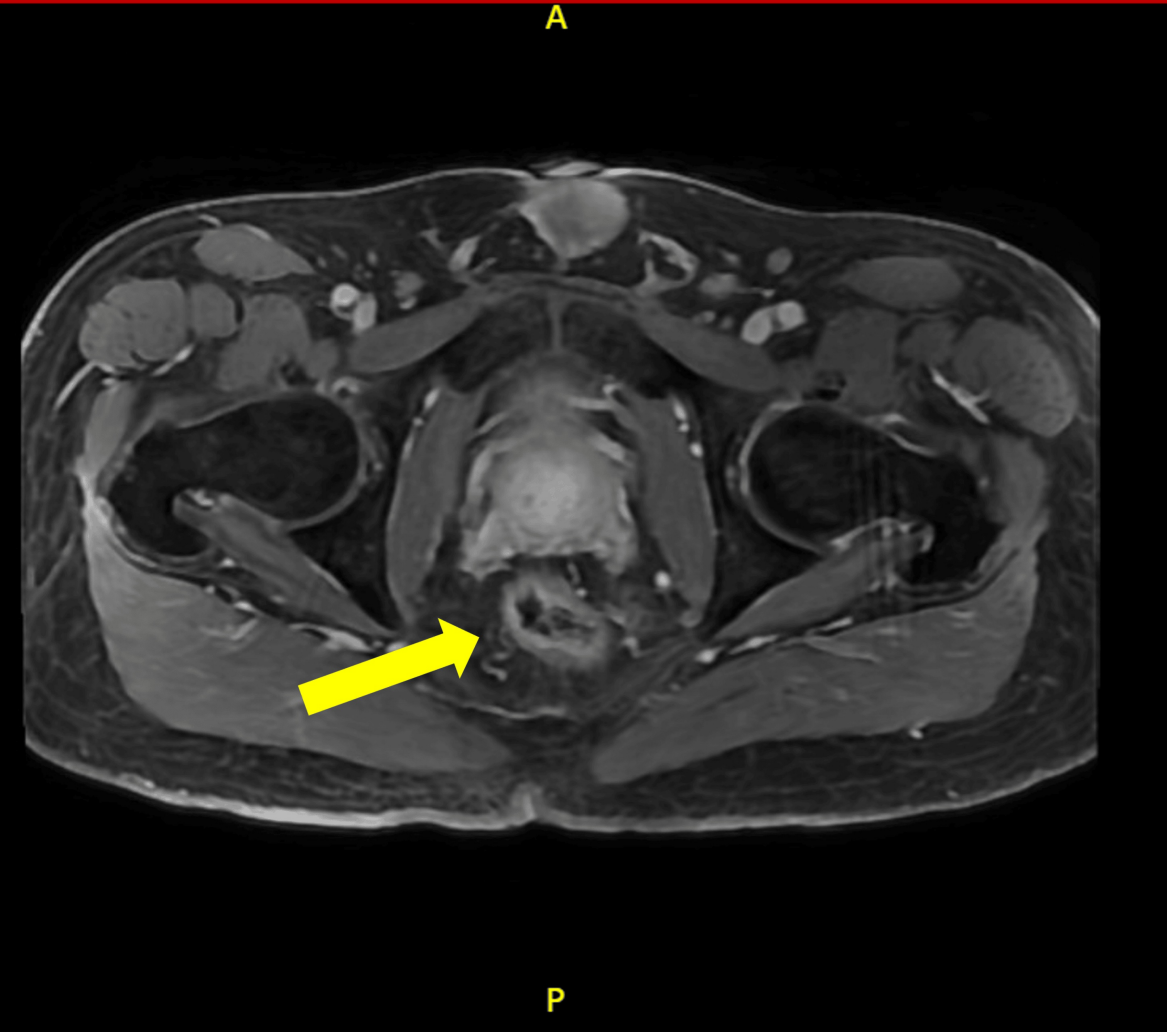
Axial T2-weighted pelvic MRI with contrast Axial T2-weighted pelvic MRI showing an intermediate signal mass involving the left aspect of the mid to lower anus (yellow arrow). The letters “A” and “P” assist with image orientation and indicate anterior and posterior, respectively.
